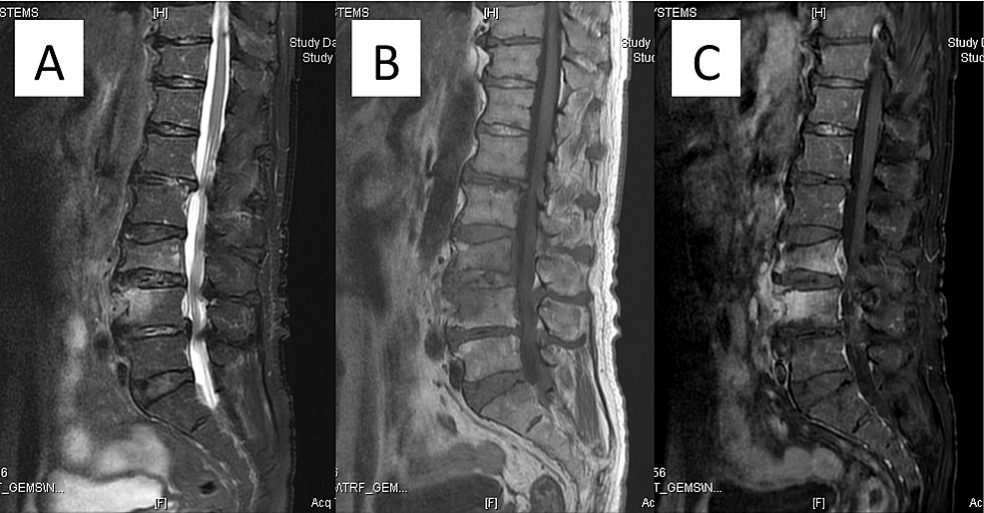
Gadolinium-diethylenetriamine pentaacetic acid (Gd-DTPA)-enhanced lumbar images obtained with magnetic resonance imaging. (A) Sagittal T2-weighted fat-suppressed image shows high-intensity changes in the L3 and L4 vertebral bodies. (B) Sagittal T1-weighted fat-suppressed image shows low-intensity changes in the L3 and L4 vertebral bodies. (C) Sagittal Gd-DTPA-enhanced T1-weighted image shows enhancement in the L3 and L4 vertebral bodies, anterior paravertebral soft tissue, and epidural abscess at the L3 vertebra level.
